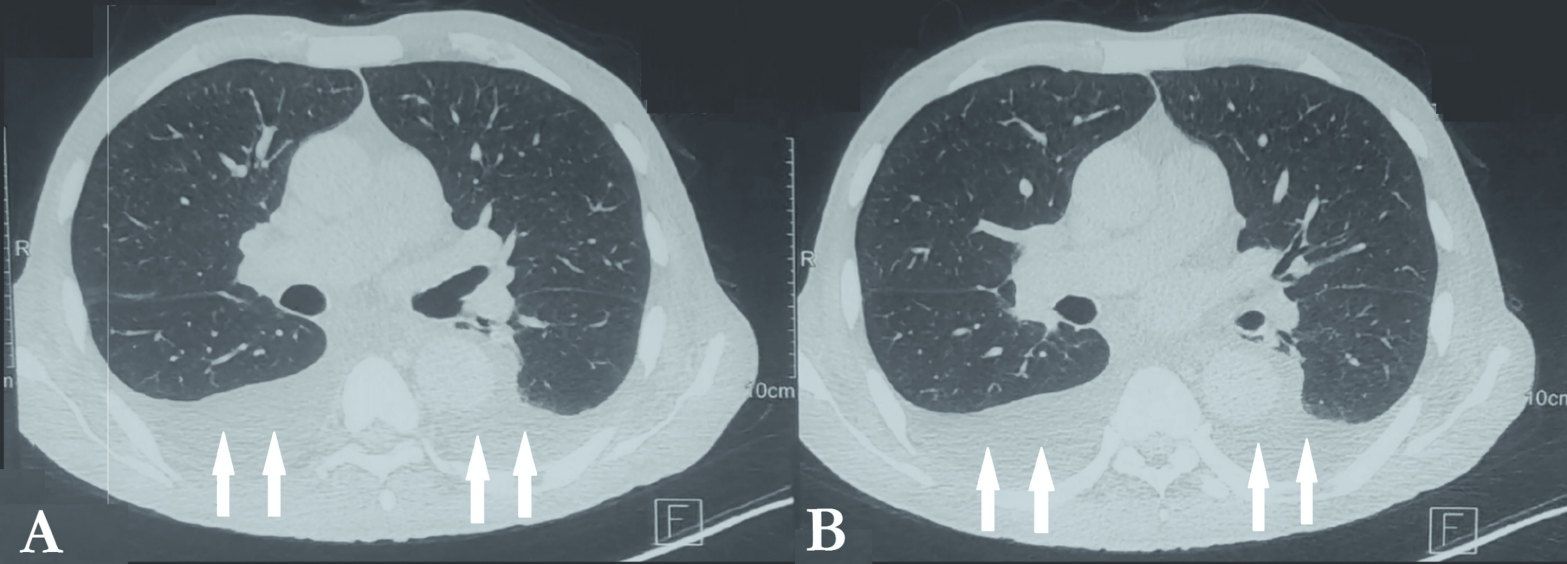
Thoraco-abdominal CT scans demonstrating moderate bilateral pleural effusion. (A) Bilateral pleural effusion. (B) Bilateral pleural effusion.
